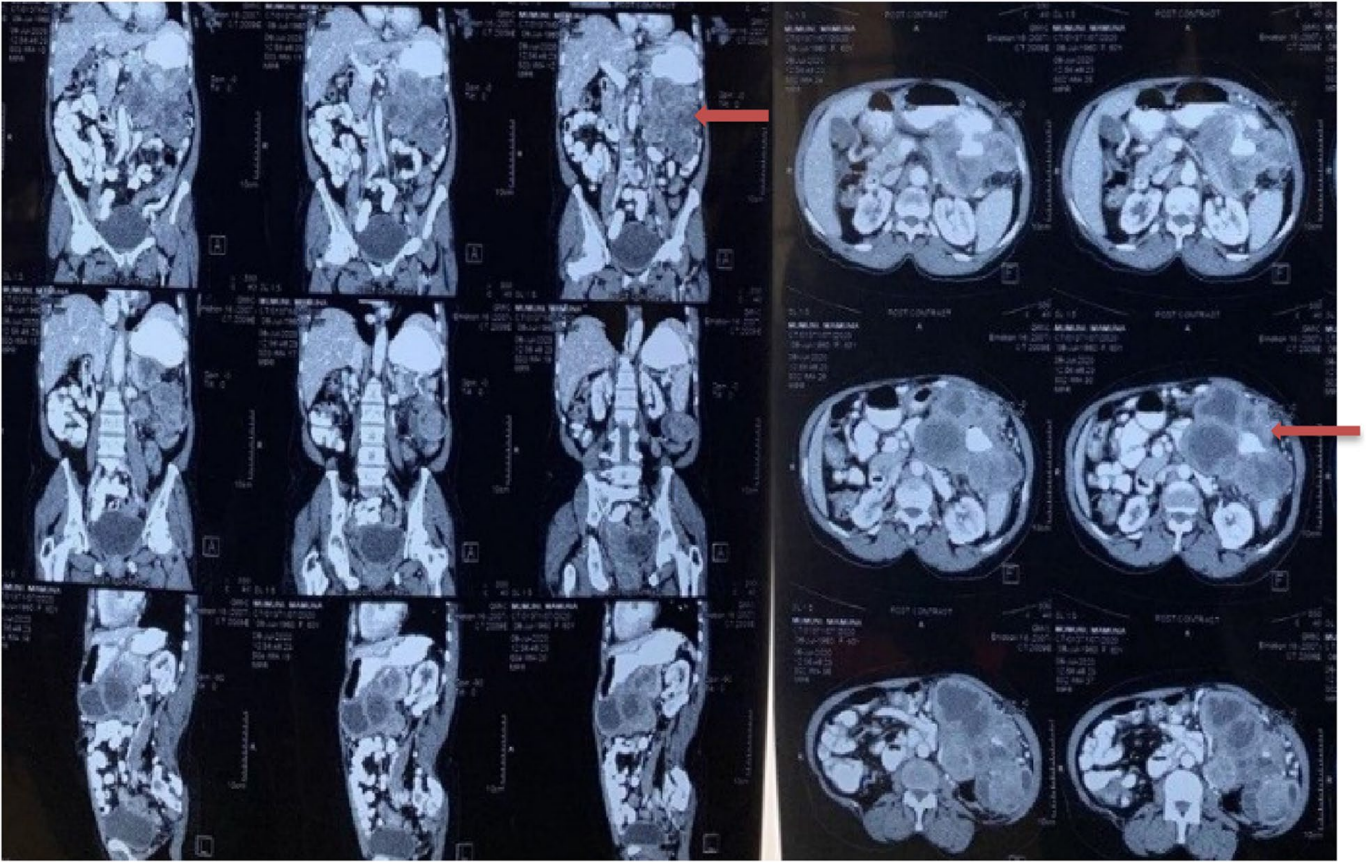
Abdominal CT scan of one patient showing mass (red arrow) originating from the stomach and invading spleen, pancreas, and small bowel
